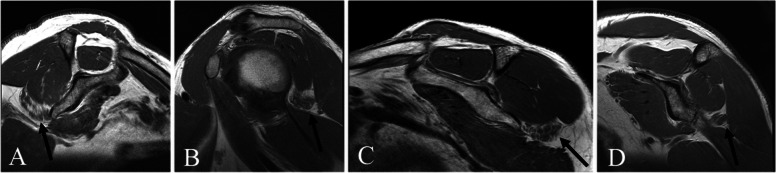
Sagittal T1-weighted MRI demonstrating fatty infiltration of the teres minor muscle in 4 patients
